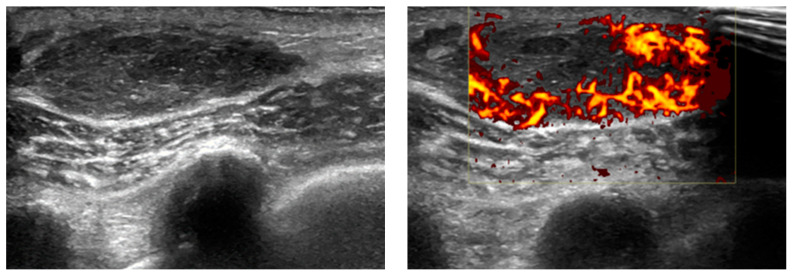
Biopsy-roven Soft tissue EP, case from our institution. Soft tissue ultrasound with B-mode and color Doppler shows a well-defined, heterogeneous, ovoid lesion with increased peripheral vascularity.
